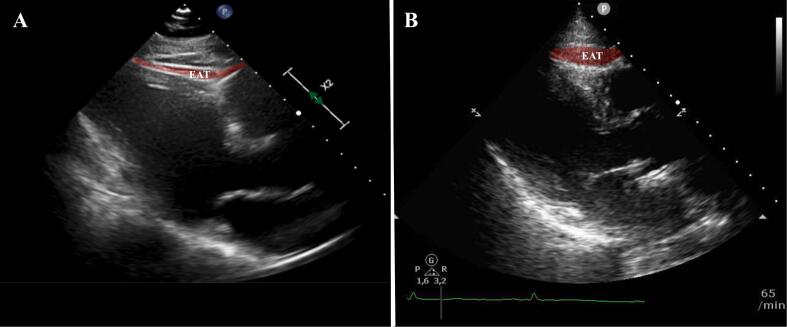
Epicardial Adipose tissue thickness in the parasternal long axis in a patient without acute COVID-19 infection (A) compared to a patient with acute COVID-19 infection (B).
